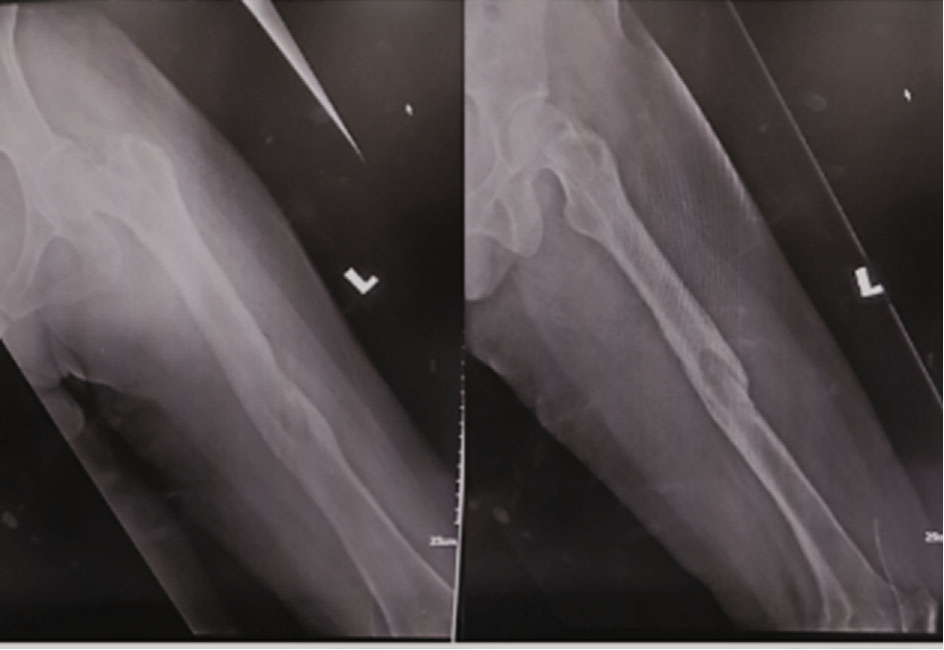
X-rays of left femur post K-nail removal.
